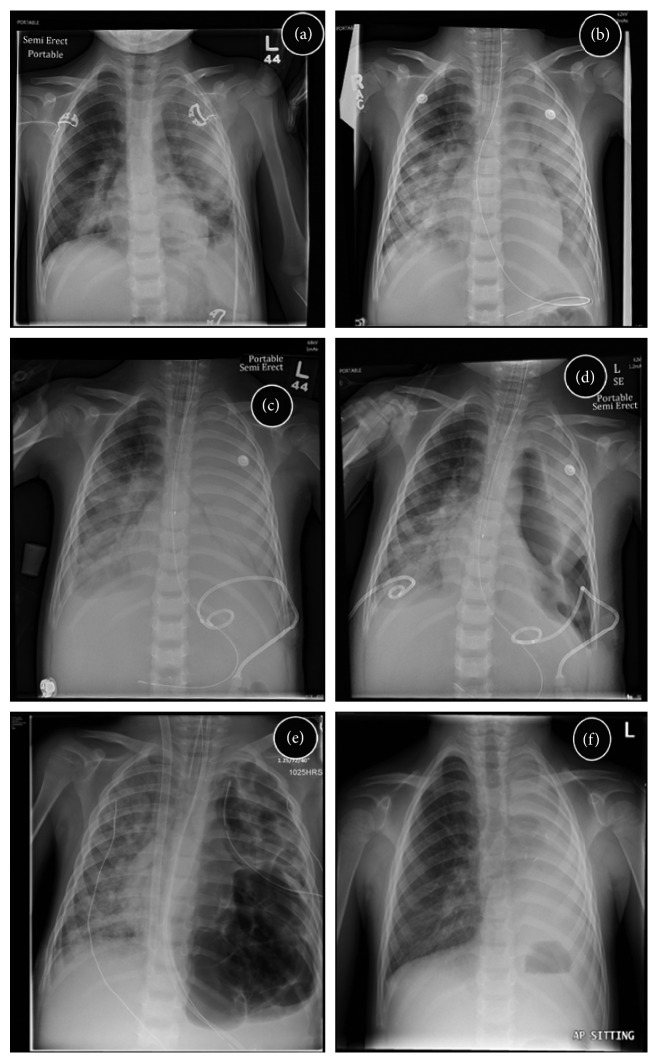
Chest radiographs showing progression of disease. (a) Presentation to ED. (b) Presentation to PICU. (c) Left hemithorax white-out, with percutaneous chest tube. (d) Right pleural effusion following drainage with the percutaneous chest tube. (e) Extensive pneumatocele development prior to pneumonectomy. (f) Resolution of acute disease, following removal of noninvasive respiratory support.
